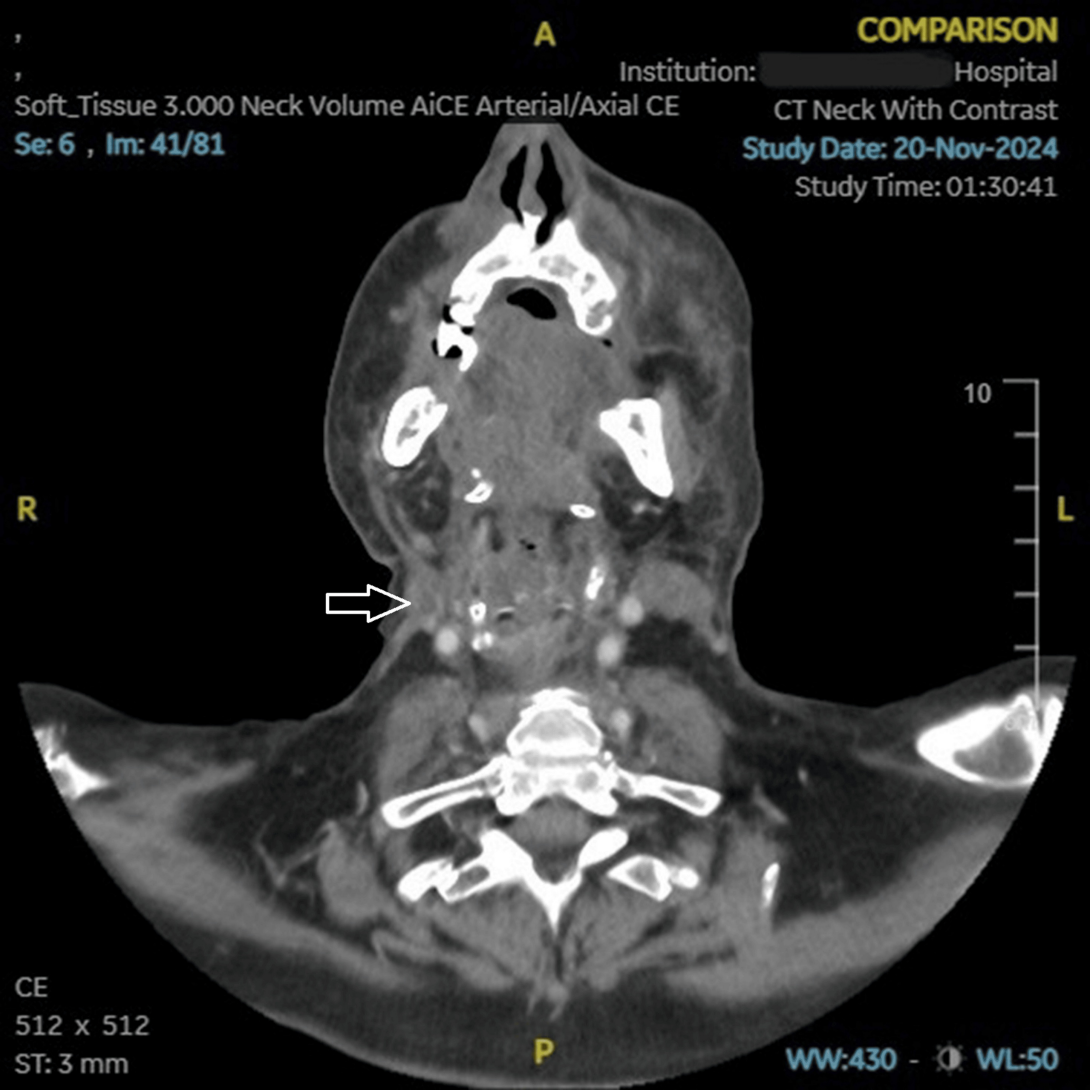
Contrast-enhanced arterial-phase CT of the neck demonstrating a non-opacified right IJV (arrow), consistent with right IJVT. The arrow points to the non-opacified right IJVT. IJVT: Internal jugular vein thrombosis, IJV: Internal jugular vein
